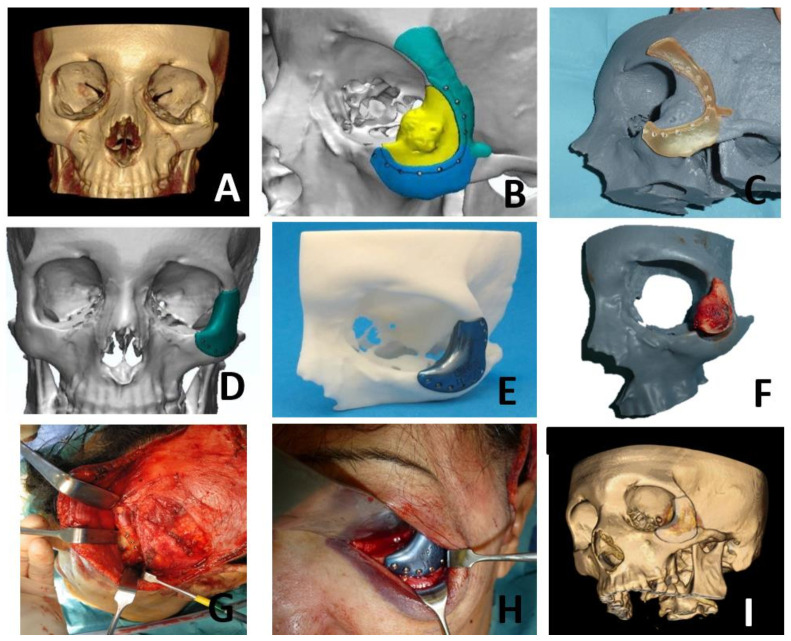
A 55-year-old woman, smoker, with a history of multiple sclerosis, reported a tumor of progressive growth and pain in the left zygomatic region. After study by CT, MRI and arteriography, the patient was diagnosed with intraosseous vascular malformation in the zygomatic bone (A). Virtual surgical planning was performed for resection and reconstruction with a titanium PSI. Cutting guides were designed for resection (B,C). A customized titanium PSI was specifically designed for the reconstruction (D,E). A hemicoronal and subciliary approach were performed and cutting guides were used to perform the resection (F–H). Once the lesion was removed, the titanium PSI was placed with an excellent fit (H). The postoperative CT scan showed a correct placement of the titanium PSI with an optimal aesthetic result (I).
